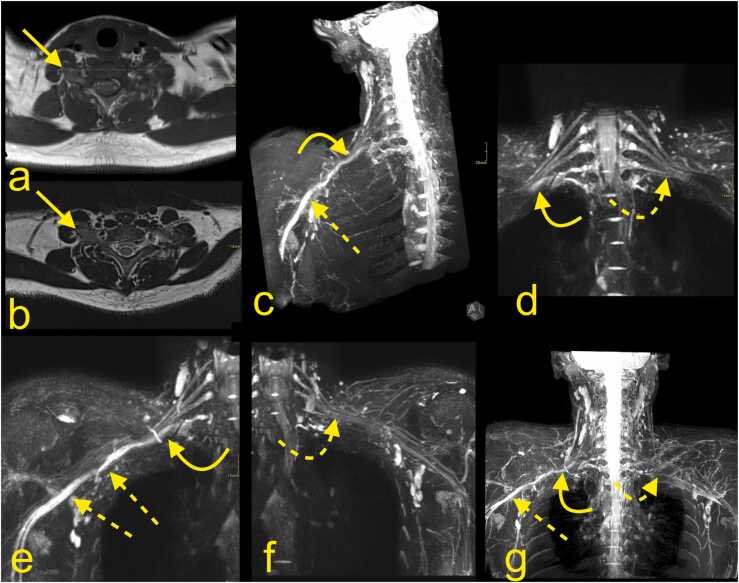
A 19-year-old patient presented with pain in the arm, forearm, and hand. A neurological examination showed a slight loss of strength in the intrinsic muscles and suspected thoracic outlet syndrome. Nerve conduction studies and EMG revealed the involvement of the lower trunk and medial cord. MRI of the BP with (a) T1-weighted TSE imaging, (b) T2 weighted TSE, and (c-g) MR neurography with (c, e, f, g) MIP reconstructions showed asymmetry of the BP (curved arrows) because of the presence of the cervical rib (straight arrow) on the right side, which is about 25 mm long. On the right side, the lower trunk (curved arrow) was displaced compared with the left side (curved dashed arrow). The medial cord and the median and ulnar nerves were swollen (straight dashed arrow).
